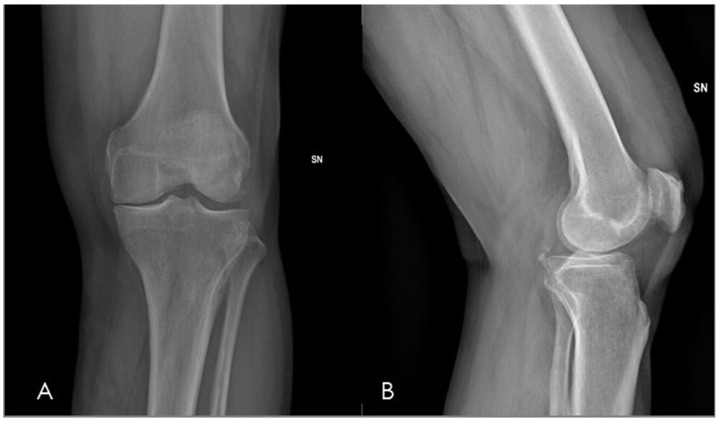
Plain radiographs of the left knee in a 70-year-old male with chronic pain refractory to conservative therapy, including anteroposterior (A) and lateral (B) views, obtained prior to GAE. The radiograph demonstrates tricompartmental osteoarthritis, characterized by joint space narrowing with associated subchondral cortical sclerosis and early marginal osteophyte formation, corresponding to Kellgren–Lawrence grade III.
